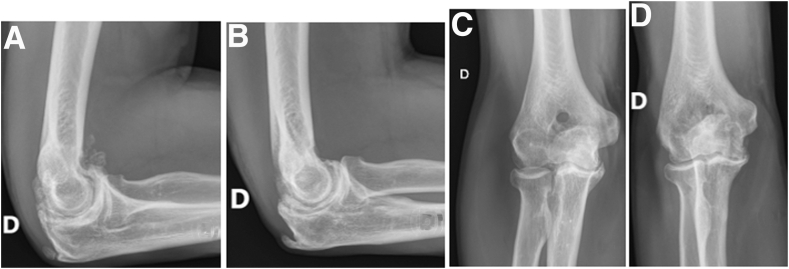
Right elbow radiographs. A lateral radiograph showing numerous osteochondromas preoperatively (A), explaining the elbow stiffness. Lateral (B) and anteroposterior (C & D) radiographs at 6 weeks and 1 year (D) respectively showed no recurrence of osteochondromas or fracture due to humeral fenestration.
